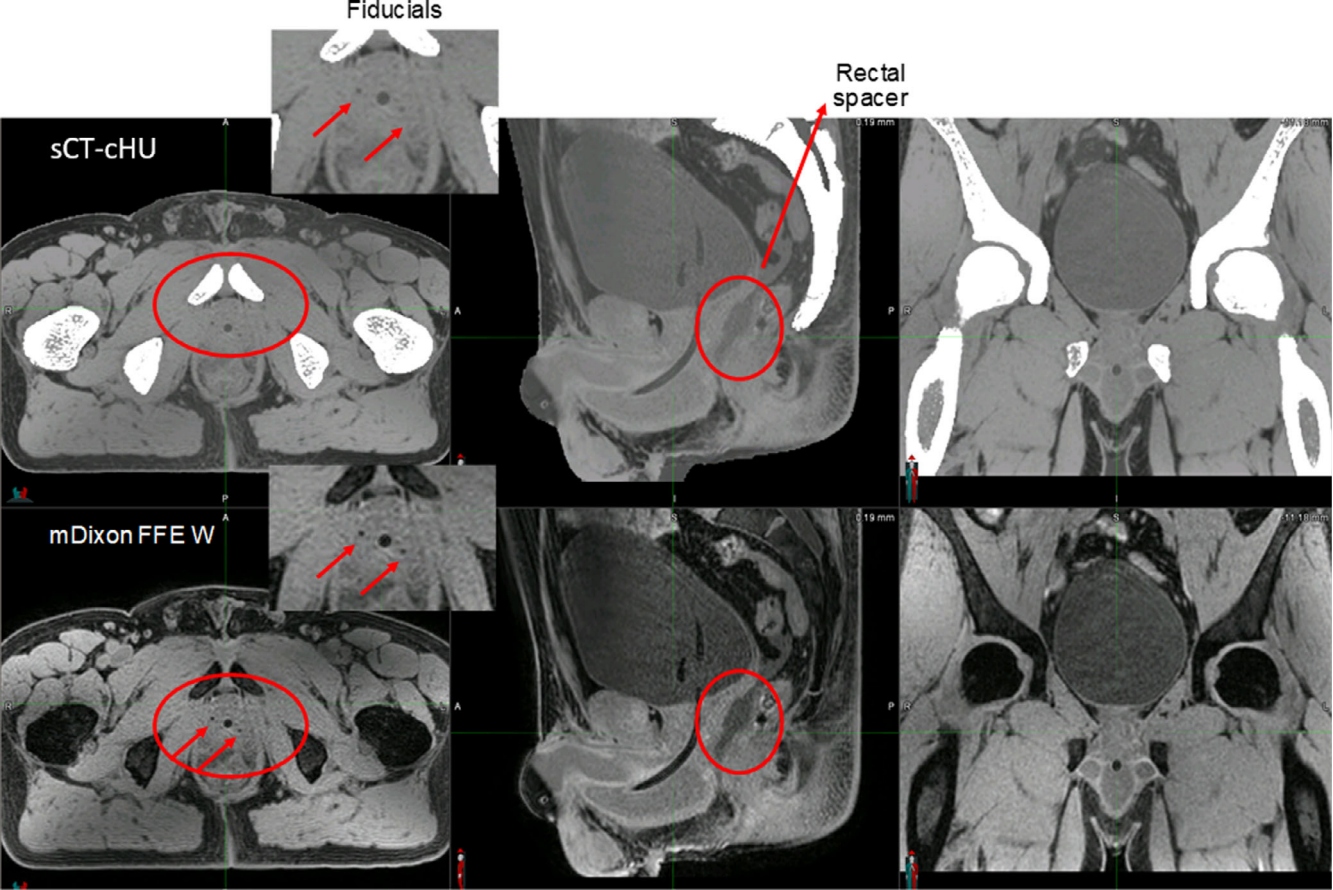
Soft tissue visibility on continuous HU synthetic CT as well as source MRI (mDIXON FFE Water). Images were acquired with 3T field strength (HU: Hounsfield unit, CT: computed tomography, MRI: magnetic resonance imaging, FFE: fast‐field echo, A: anterior, P: posterior, S: superior, I: inferior).
